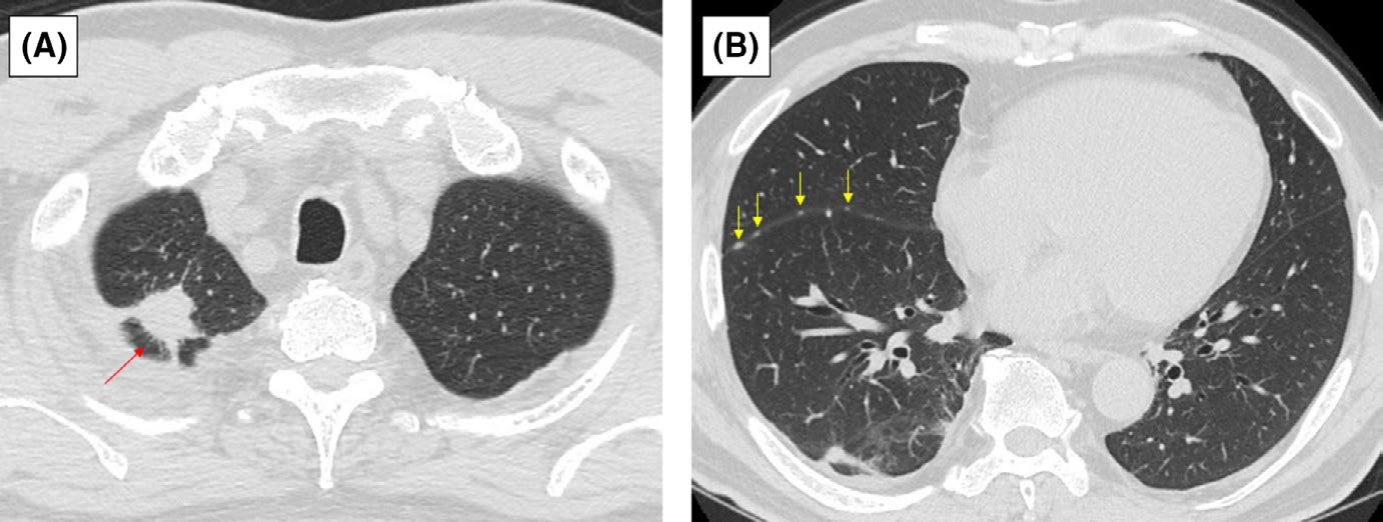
Radiological findings of the patient. High‐resolution computed tomography (HRCT) shows a spiculated 12‐mm nodule (A, red arrow) in the right upper lobe, and fine granular shadows (B, yellow arrows) on the right indicate major fissure. In contrast, right‐sided limited pleural effusion is observed (A, B)
